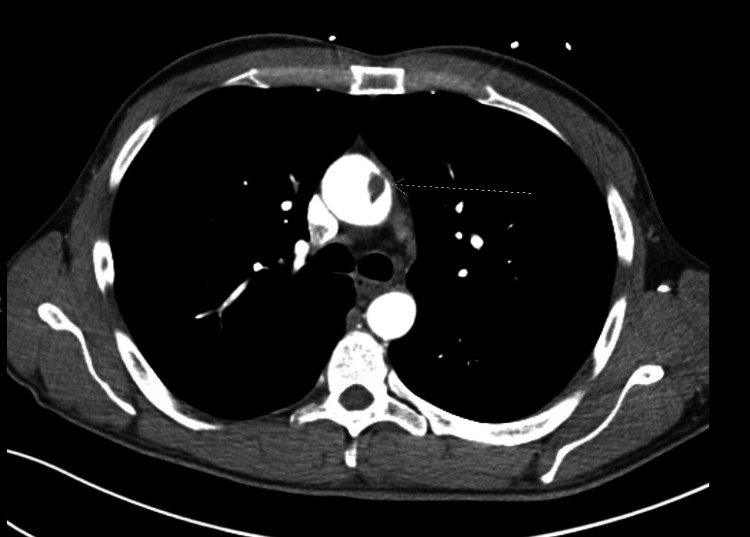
CT angiography of the ascending aorta with the white arrowing pointing at the large 15mm free-floating thrombus
